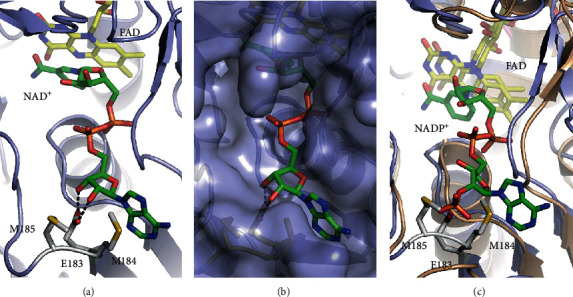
Table 1 tab1: X-ray data collection and refinement statistics for *Af*Npsr.
